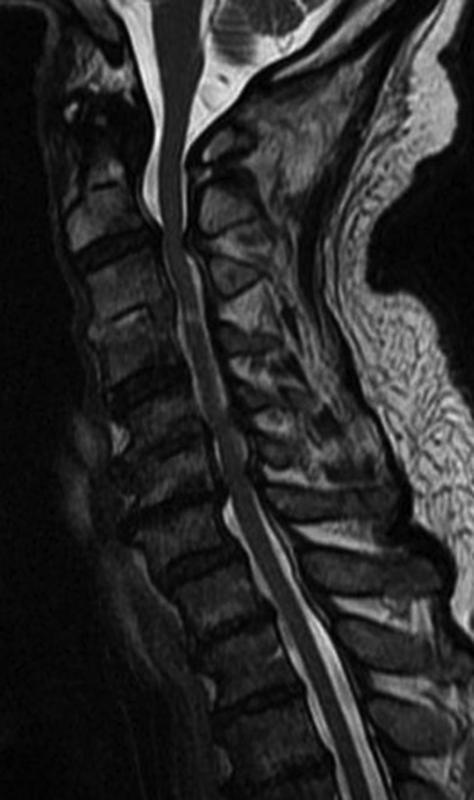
Preoperative magnetic resonance image.
